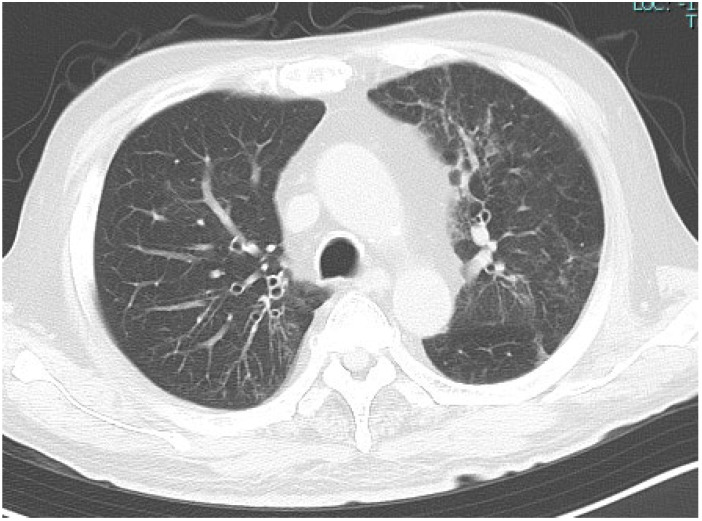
Chest CT on day 28. Chest CT showing improvement in the pneumonia findings. CT, computed tomography.
